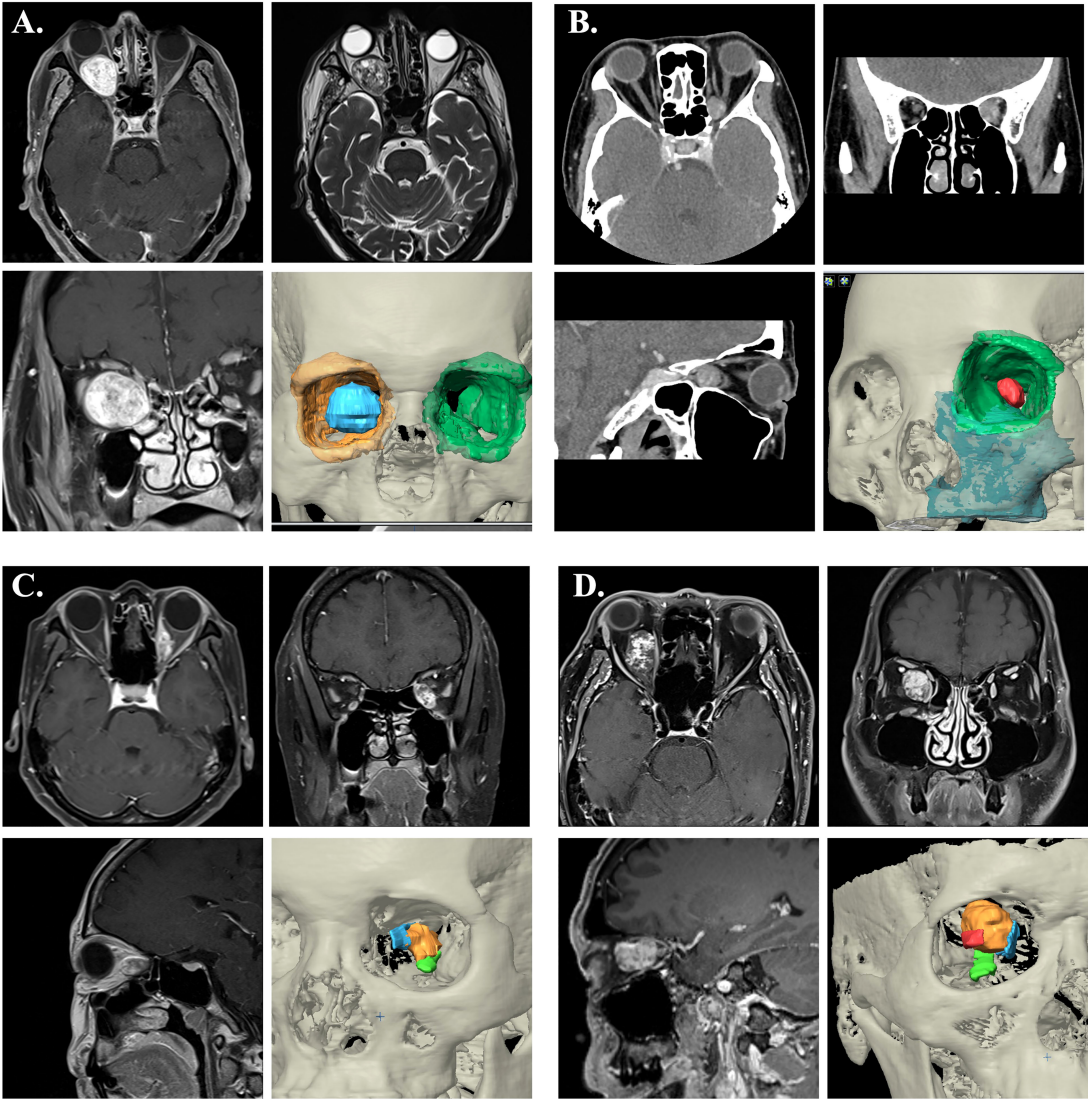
Image characteristics of the cases. (A) The contrast-enhanced MRI showed a retrobulbar intraconal well-demarcated ovoid mass of right orbit. The tumor showed heterogenous appearance on T2W sequences with some internal cystic component, and significant contrast enhancement. The tumor deviated the right optic nerve upward and causing right proptosis. 3D image rendering tumor lesion was demonstrated (blue). (B) The CT showed an ovoid, well-defined with contrast-enhanced mass at the medioposterior extraconal space and medial to the apical region of the optic nerve. 3D image rendering tumor lesion was demonstrated (red). (C) The MRI demonstrated an enhancing mass at medial side of left intraconal space (orange), which involving the medial rectus muscle (blue) and superior to inferior rectus muscle (green). (D) The MRI revealed a heterogeneously enhancing mass lesion at the right intraconal space (orange), medial to the optic nerve (red) that displaced laterally. (Blue: medial rectus muscle; Green: inferior rectus muscle)
